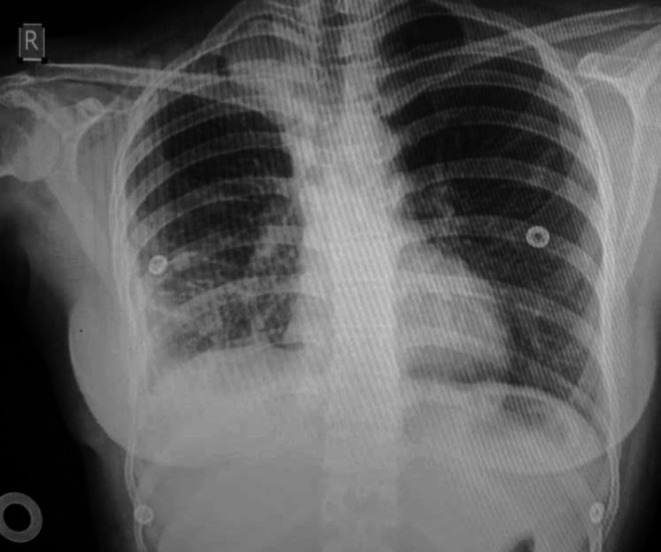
Postoperative chest radiograph (portable AP view) shows improved right lung expansion.
